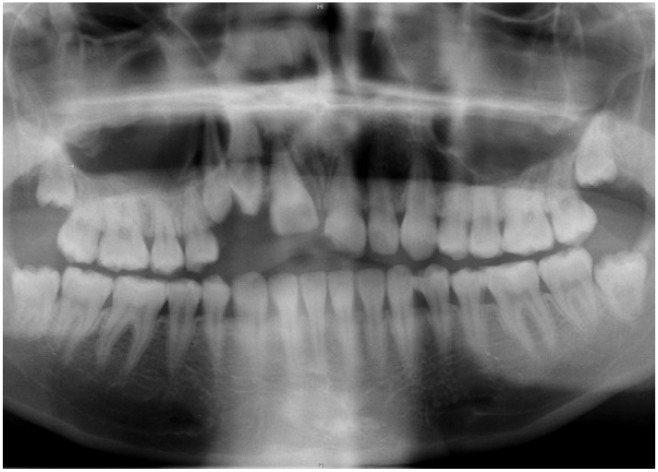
Panoramic radiographic investigation directly after trauma (Image: Institute of Radiology, Kantonsspital Aarau, AG, Aarau, Switzerland).
